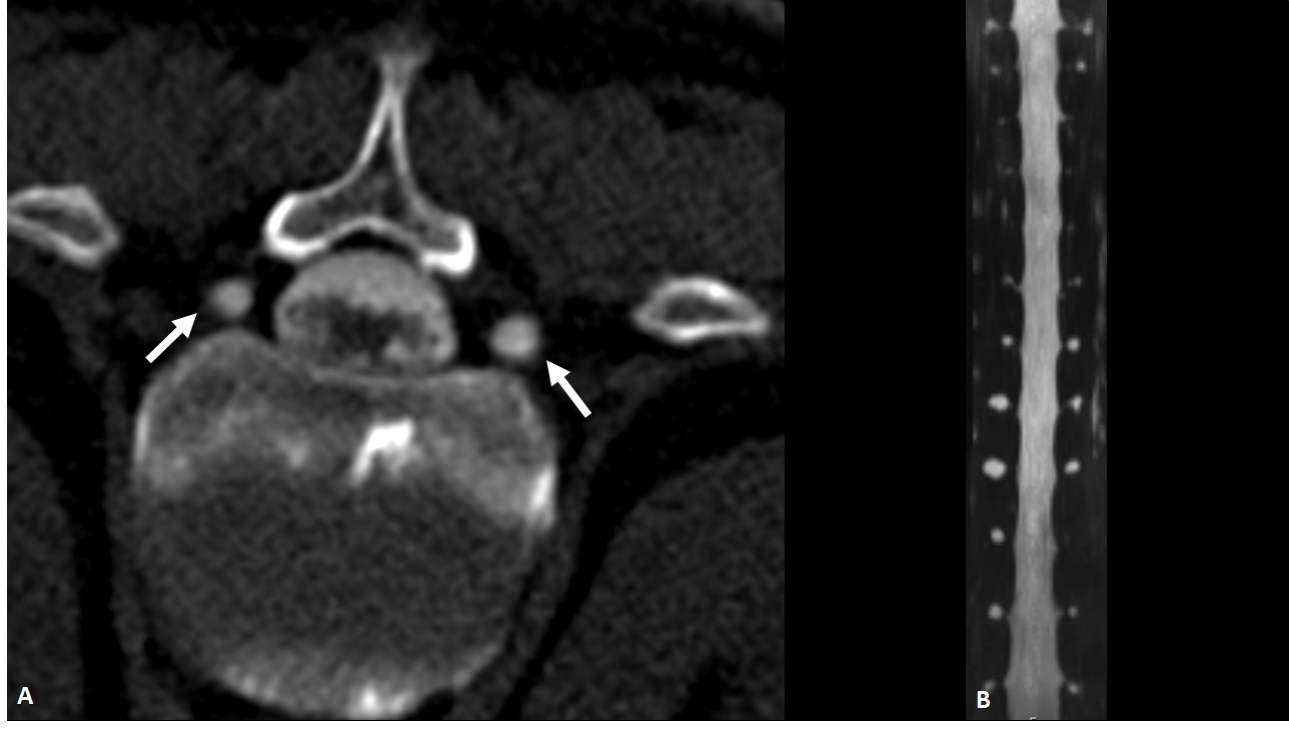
CT Myelogram CT myelogram in (A) axial and (B) coronal reconstruction demonstrating bilateral small perineural (Tarlov) cysts of the spine at multiple levels (white arrows).
